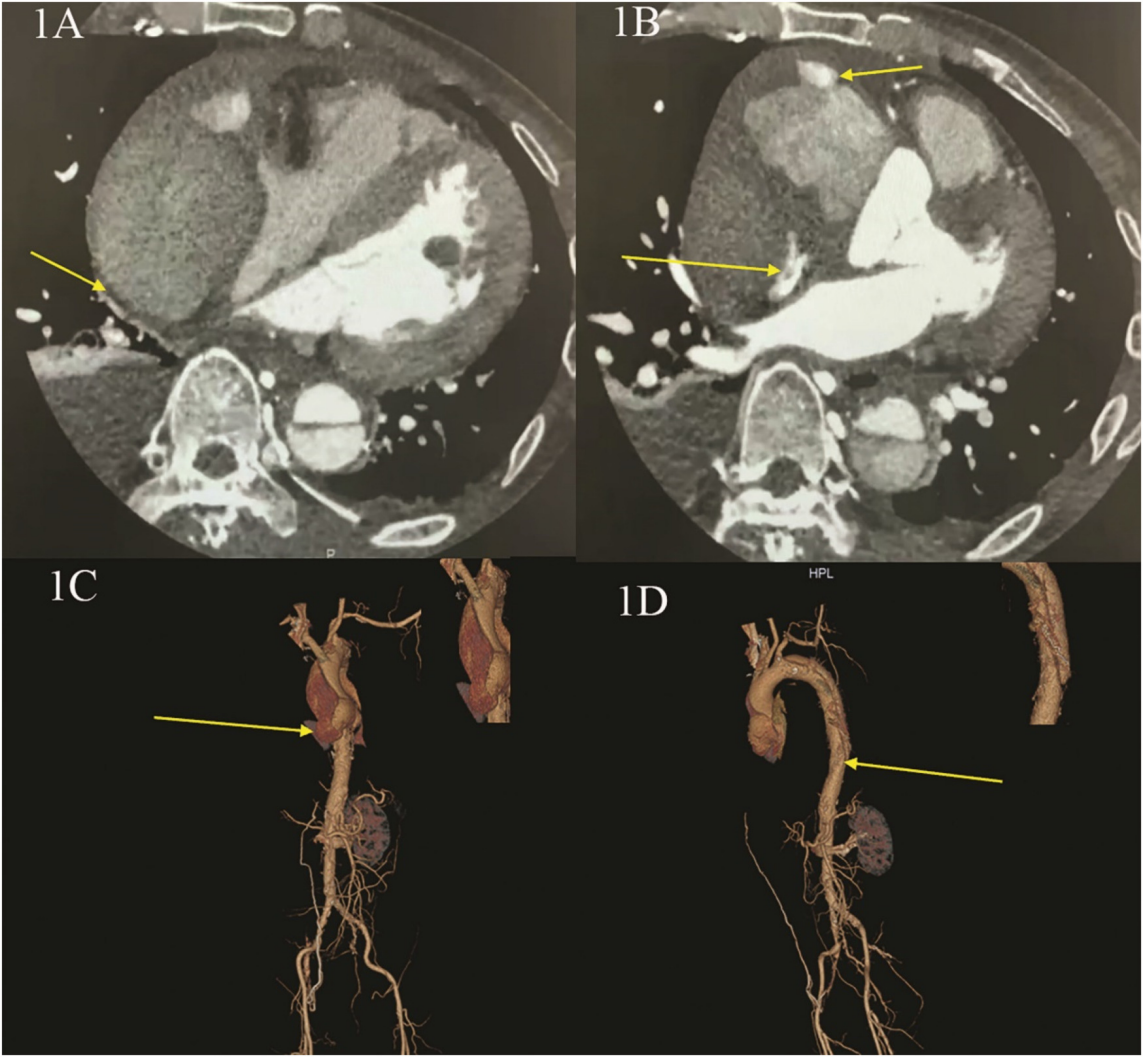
Preoperative CT angiography (CTA) of the thoracic aorta and abdominal and coronary arteries. (A,B) CTA shows: entrapment with aneurysm rupture in the root of ascending aorta, forming a huge pseudoaneurysm and thrombus, with obvious compression of the right heart; (C,D) CTA three dimensional reconstruction shows: the first rupture of aortic entrapment is located in the root of the ascending aorta, and the second rupture is located in the descending aorta.
